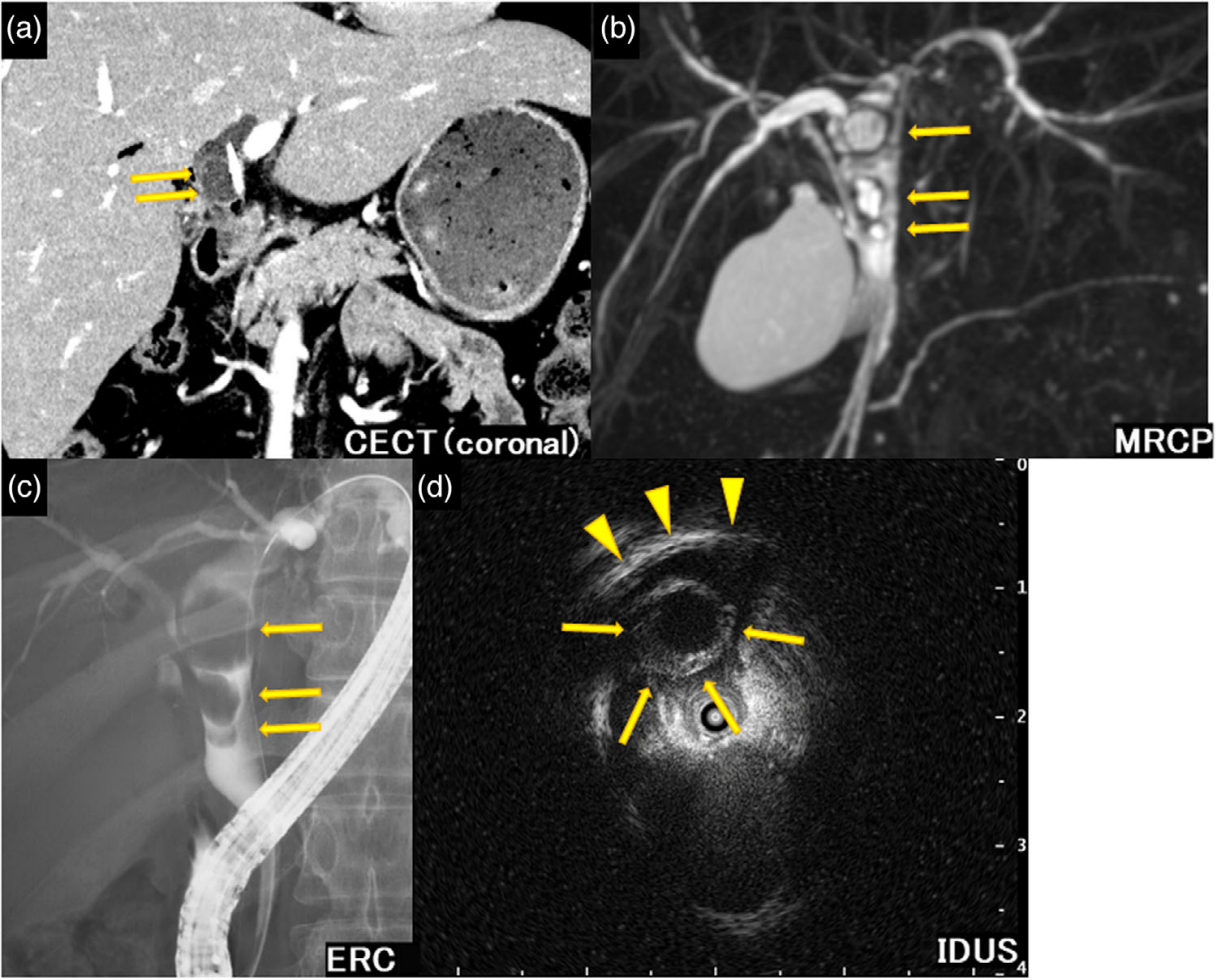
Radiological findings of the extrahepatic bile duct lesion. (a) Contrast‐enhanced computed tomography (CECT) revealed a 20 mm cystic lesion with a thin external capsule in the common hepatic duct. (b) Magnetic resonance cholangiopancreatography (MRCP) showed strong hyperintensity in the extrahepatic bile duct on T2 weighted imaging, suggesting that the lesion mainly consisted of a cystic component. (c) Endoscopic retrograde cholangiography (ERC) showing diffuse bile duct dilation and three round contrast defects in the extrahepatic bile duct. (d) Intraductal ultrasonography (IDUS) shows anechoic lesions with a 2–5 mm low echoic external capsule (arrow); however, the bile duct wall is not thickened (arrowhead).
